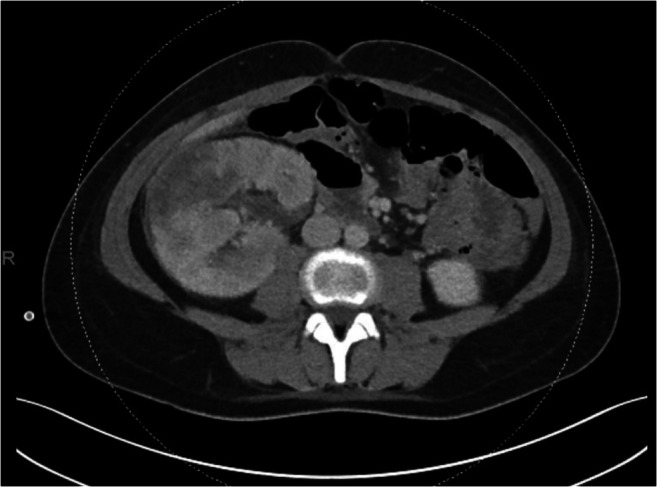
CT scan without contrast, right after the patient had developed fever. (1) Decreased volume of pyelum/hydronephrosis compared to the previous CT scan. (2) Increased area of lower density in the renal parenchyma, suspect for extension of pyelonephritis as tissue becomes increasingly hydrous. (3) Resorption of the blood clot in the proximal ureter. There are no signs of an encapsulated abscess
